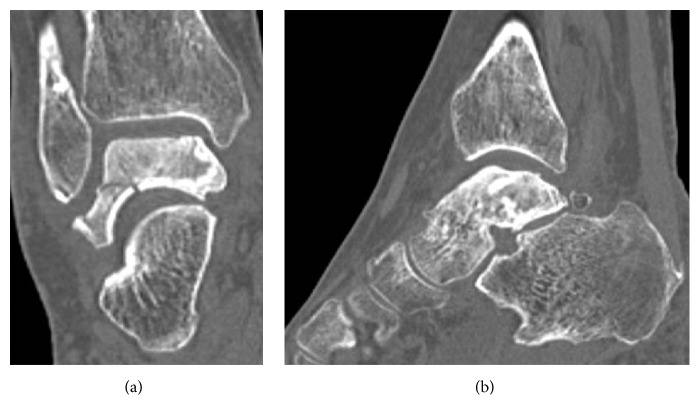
Preoperative CT scans showing sclerotic change and collapse of the talar body in coronal (a) and sagittal (b) views.
